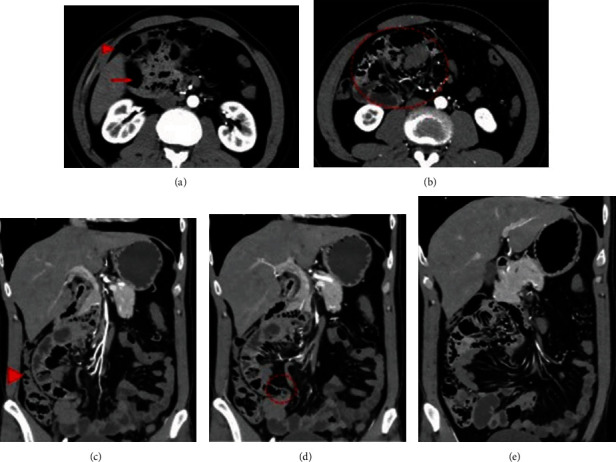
CT scan in Case 1. (a) There was no duodenal horizontal section. The duodenal descending section and jejunum (arrow) were located under the ascending colon (arrowhead). (b) The jejunum (dotted area) was located in the right upper quadrant. (c) The ascending colon (arrowhead) was recognized at the normal position. (d) The part where the jejunum excluded to the retroperitoneal side comes out into the abdominal cavity was narrowed like a “hernia defect” (dotted area). (e) The small intestine that emerges into the abdominal cavity runs to the left and then reached the ileocecal region.
